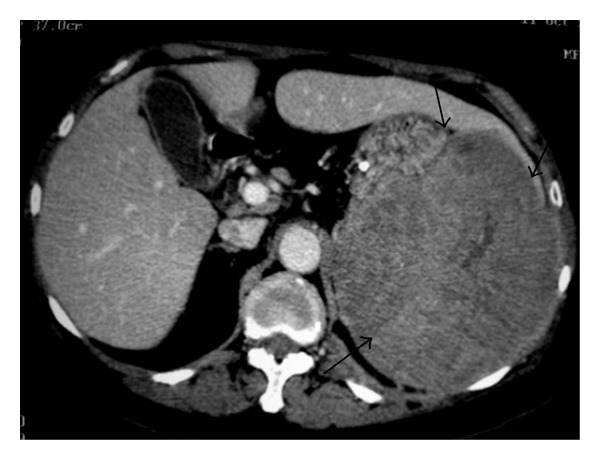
NHL involving the spleen. Axial abdominal CT after administration of intravenous CM reveals a large mass in spleen (arrows).
